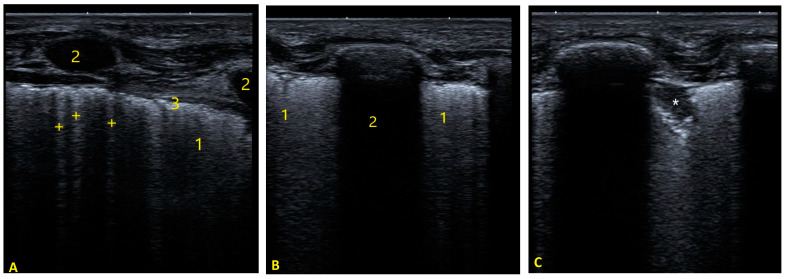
Linear transducer lung ultrasound images showing: (A) Left-side panel: B-lines. In this image, multiple B-lines (+) are observed in the intercostal space, some coalescing, giving rise to a white lung image. Irregular and poorly defined pleuropulmonary line. (1) Coalescing b-lines, white lung, (2) ribs and (3) pleuropulmonary line. (B) Central panel: Coalescing b-lines or white lung (1) in two adjacent intercostal spaces delimited by a rib (2). (C) Right-side panel: Subpleural consolidation. Hypoechoic image with frayed edges (*).
